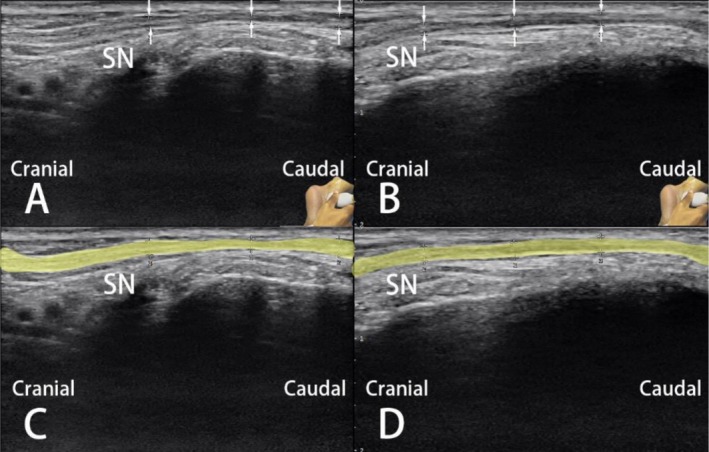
Musculoskeletal ultrasound examination images. (A, C) Long‐axis images of the sural nerve (SN) before the first treatment (November 12, 2025); (B, D) Long‐axis images of the nerve after the first treatment (November 22, 2025).
